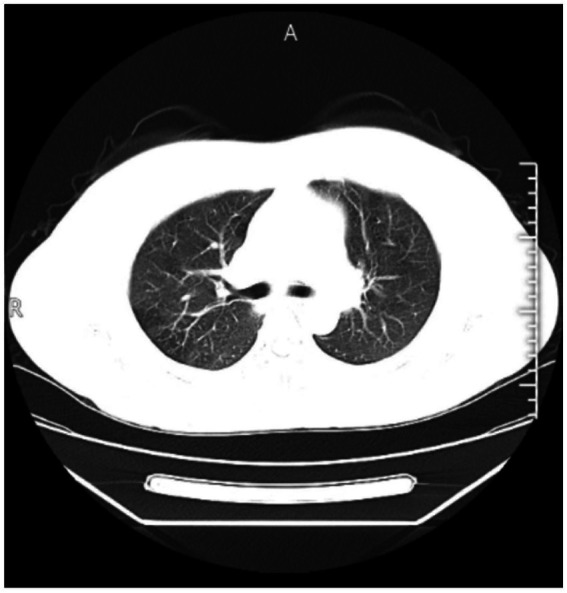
Chest CT scans showed no significant abnormalities.
